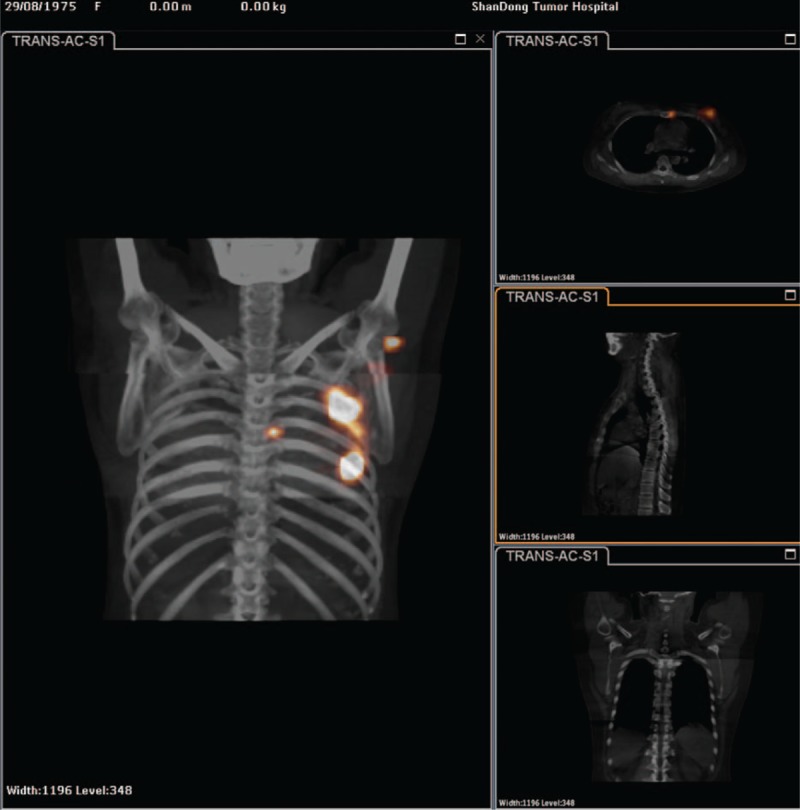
Preoperative lymphoscintigraphy revealed that there was a “hot-spot” in third intercostal space.
